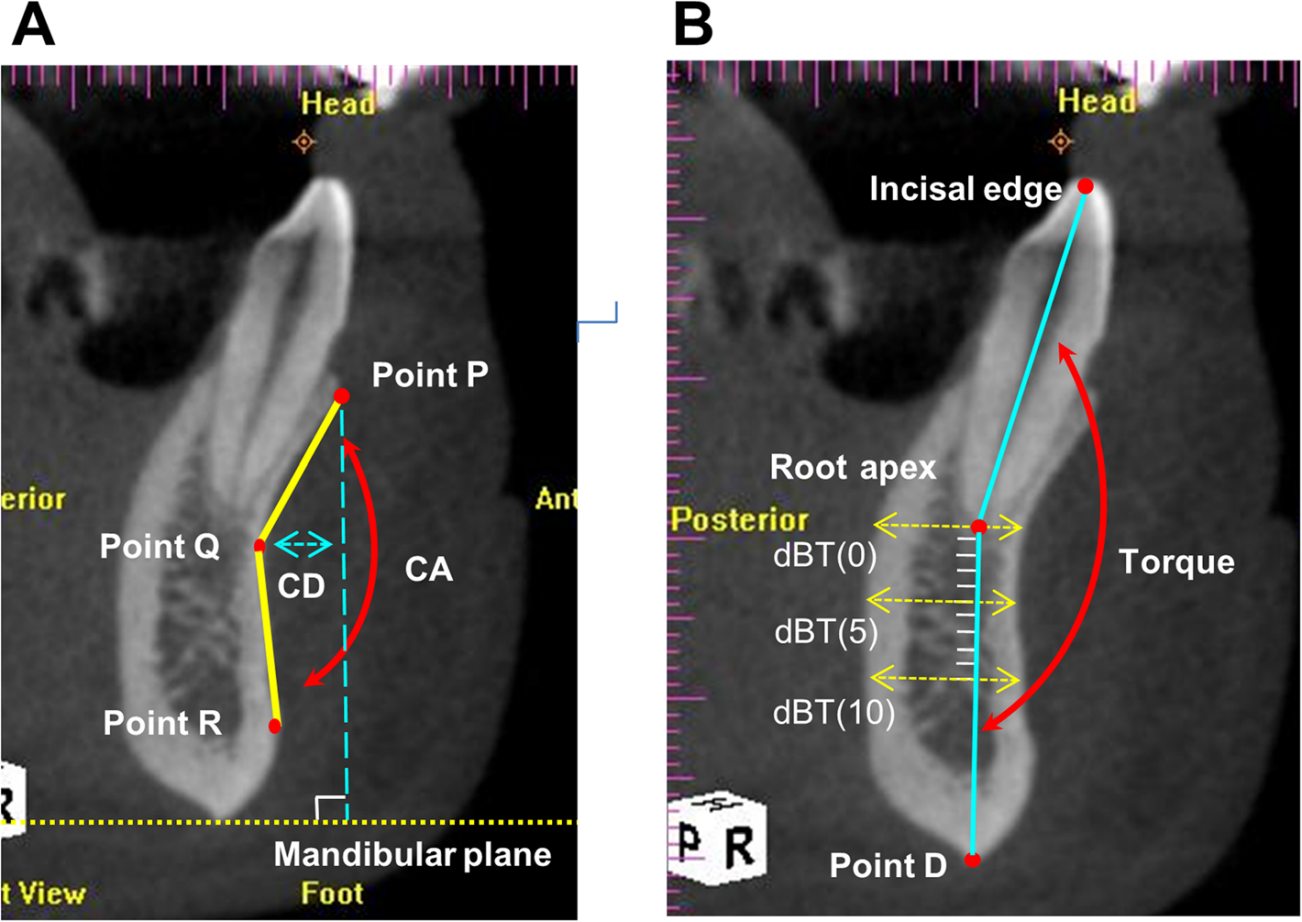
Anatomical parameters regarding labial bone perforation (LBP) of the anterior mandibular region in sagittal radiographic images. a Landmarks and anatomical parameters of the concavity depth (CD), and concavity angle (CA). b Landmarks and anatomical parameters of the torque (T), and deep bone thickness (dBT). Each measurement line of dBT was also perpendicular to the mandibular long axis, and then the distance between the facial and lingual outline of the bone was measured
